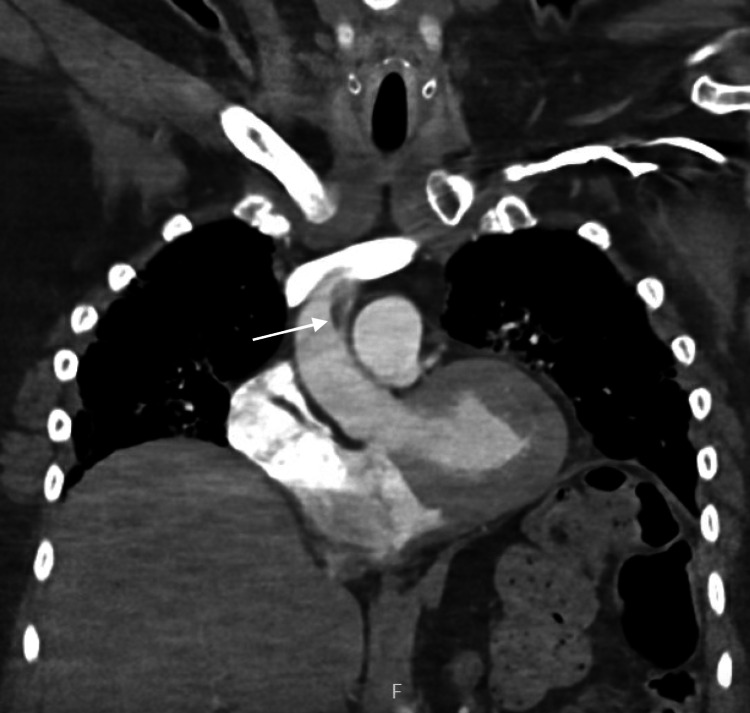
Ten-day interval chest CTA demonstrating a decrease in size of the aortic intraluminal filling defect
